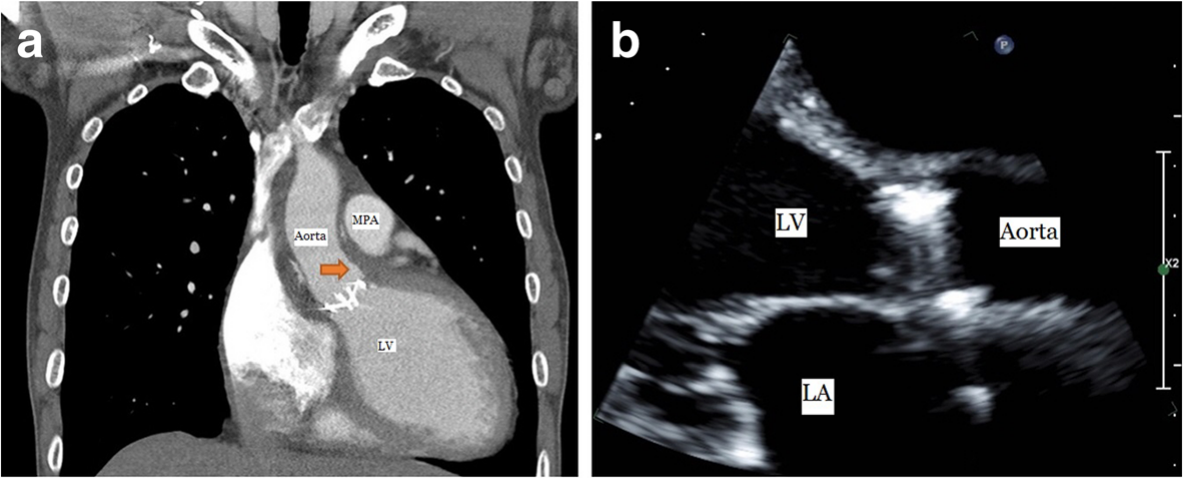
a Postoperative chest computed tomography shows that pseudoaneurysm of sinus of Valsalva is only a trace left (arrow). b Transthoracic echocardiography shows that the sac in left ventricle disappeared. MPA, main pulmonary artery; LV, left ventricle; LA, left atrium
